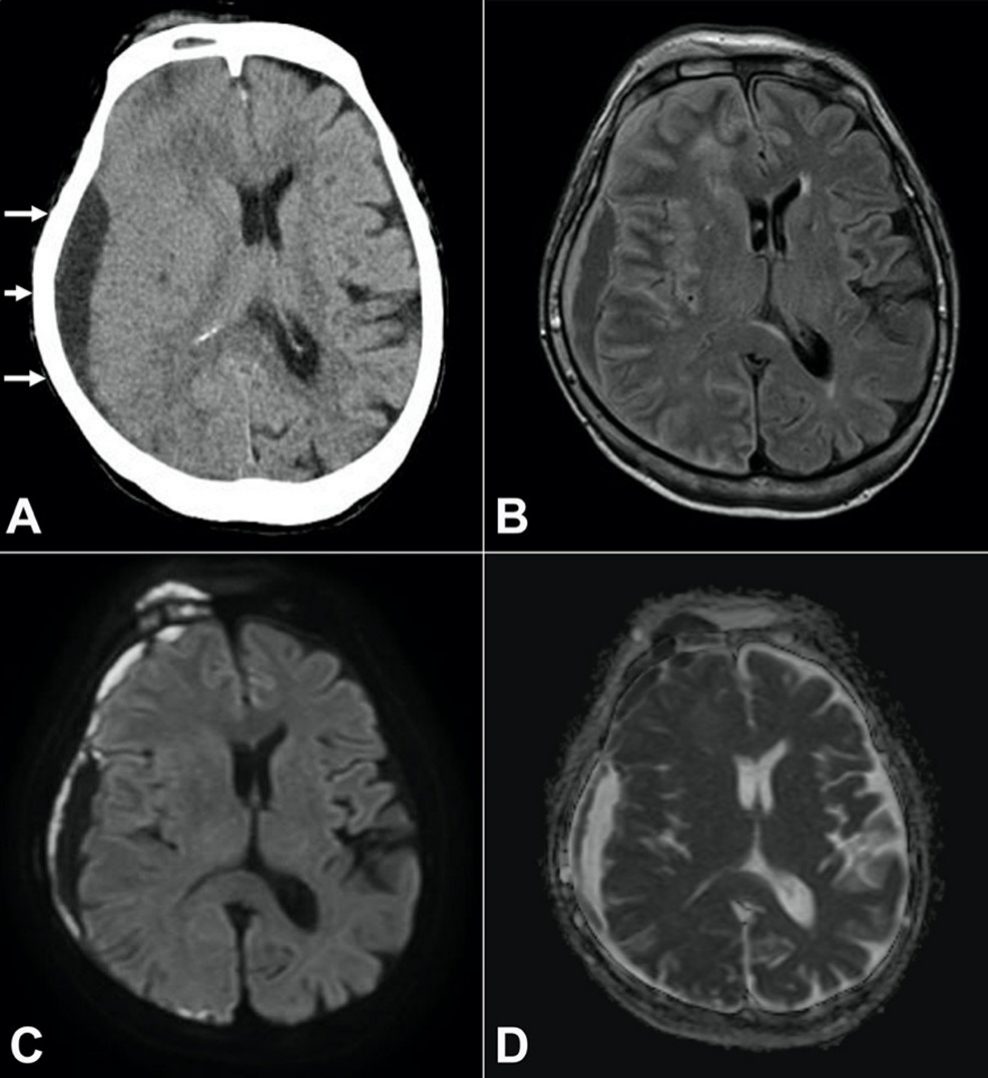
Head CT (A) and MRI (B-D). A - A CT scan showed a hypodense crescentic collection in the subdural space (arrow) with a mild mid-line shift. A swollen mass was also seen on the right frontal head; B - A FLAIR image showed low signal intensity in a crescentic formation surrounded by a peripheral hyperintense rim; C - DWI showed that the crescentic cavity consisted of internal hypointensity and linear hyperintensity of the dura mater extended to the right frontal contusion site. The subcutaneous mass on the right frontal head, an adjacent frontal bone, and lobe also showed a restrictive pattern, most likely indicating a subdural hematoma accompanied by bone and brain contusions; D - The ADC value of the central area of the crescentic collection was high, whereas its peripheral region showed a low ADC value.
